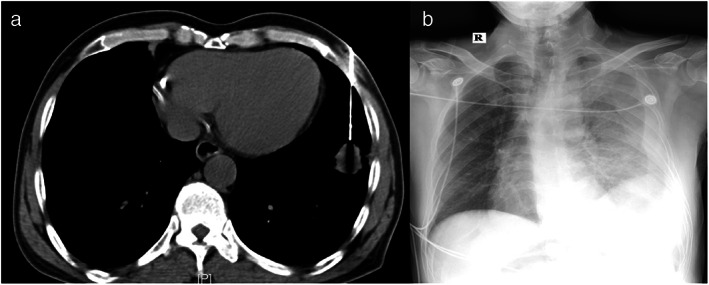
Depicts the case of a patient with severe hemothorax after computed tomography (CT)‐guided lung puncture. A 56‐year‐old patient was found to have a nodule in the left lower lobe identified on CT imaging, and the nodule was sampled by lung puncture. Because of the longer surgical path, the biopsy procedure was more complex and lengthier, leading to the development of progressive hemothorax
